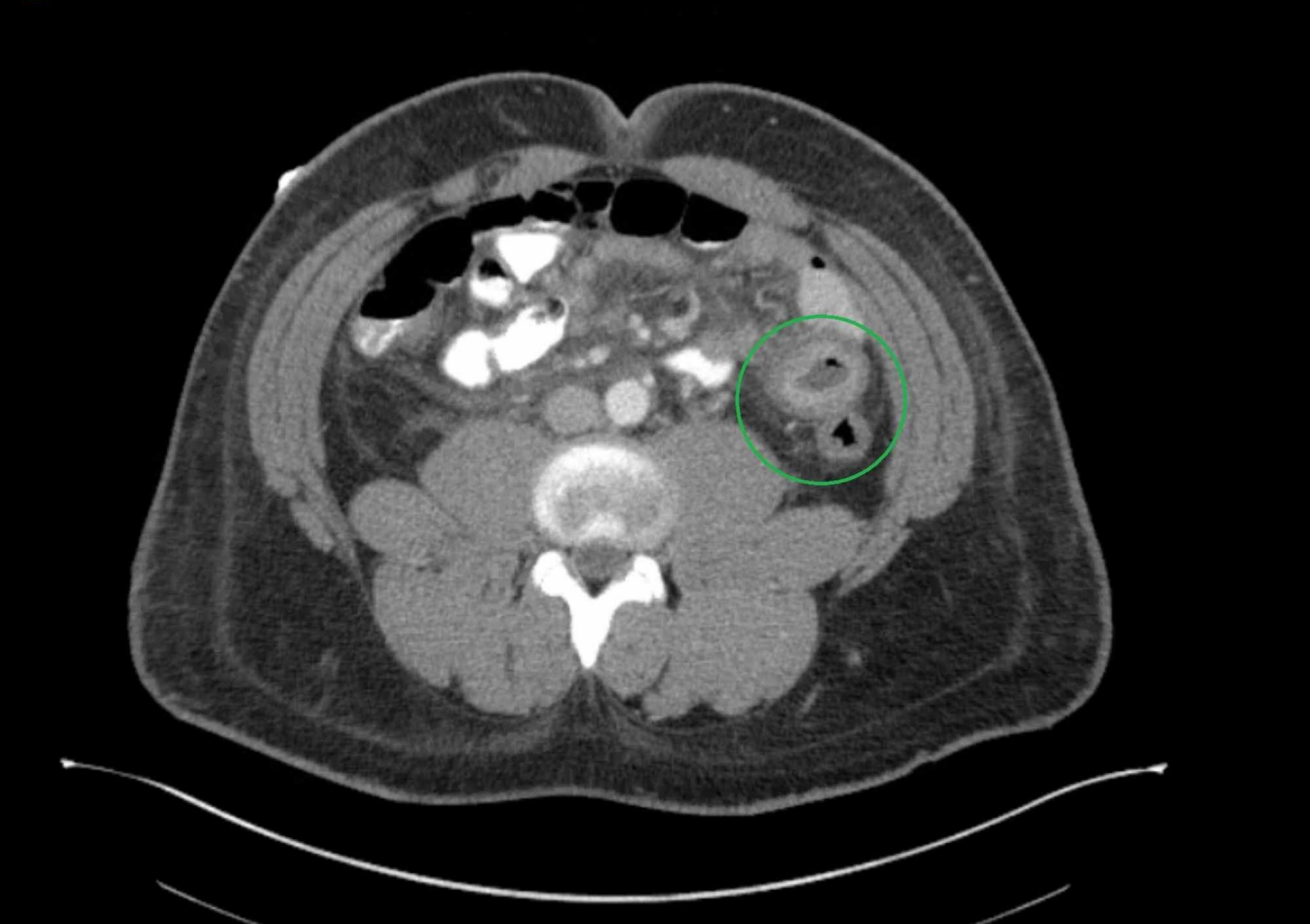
Target sign on CT of the abdomen (green circle) CT: computed tomography
